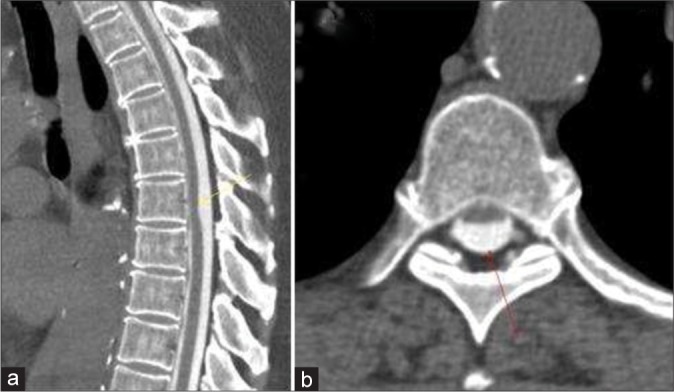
A 67-year-old female who presented with complains slowly progressing bilateral leg weakness with associated chronic low back pain. (a) Sagittal and (b) Axial CT Myelogram images of the thoracic spine demonstrating anterior cord kink at T6 (Yellow arrow) with complete opacification of CSF posterior to the anteriorly displaced spinal cord (Red arrow).
